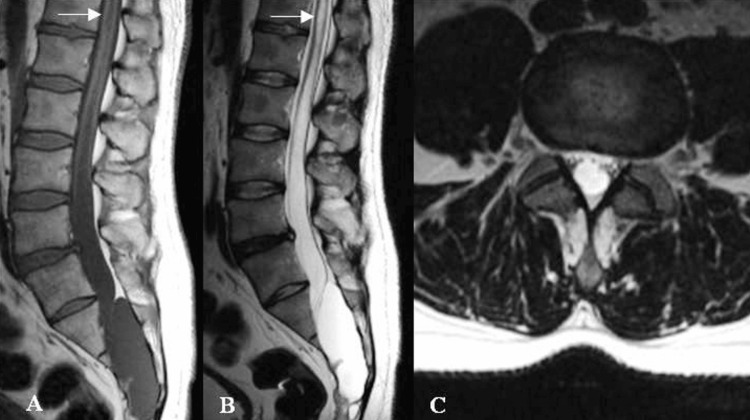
MRI of an intradural arachnoid cyst at the level of S1-S5. (A, B) Sagittal projections at T1 and T2 demonstrate cystic formation extending from the cranial surface of the S1 vertebra to S5 with liquor-signal intensity-hypointensity at T1 and hyperintensity at T2. (C) Axial projection shows the compressed nerve roots of the cauda equina.
